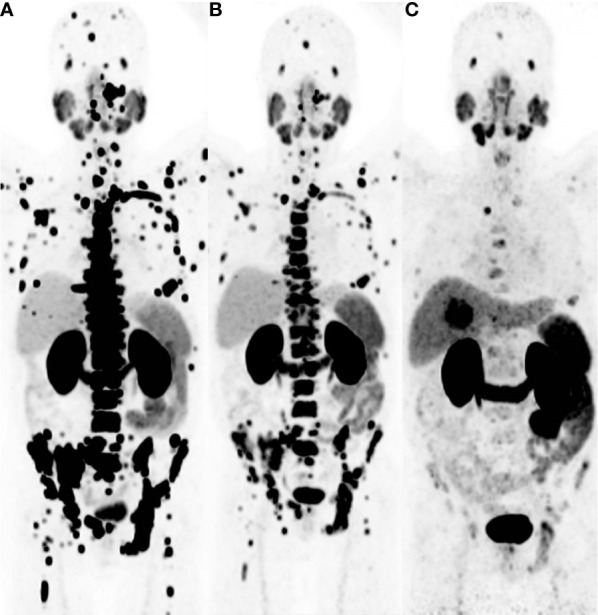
This 63-year-old male patient was diagnosed with prostate cancer 6 years ago and underwent a ^68^Ga-PSMA PET/CT imaging examination. The maximum intensity projection (MIP) image revealed a significant increase in tracer uptake in the skeletal region (A). The patient received 4 cycles of ^225^Ac-PSMA-617 therapy, and after 2 treatment cycles, subsequent ^68^Ga-PSMA PET/CT imaging still showed multiple areas of tracer uptake in the skeletal region, despite a notable reduction in SUVmax (B). A ^68^Ga-PSMA PET/CT imaging performed 16 weeks after the last treatment showed a substantial decrease in tracer uptake in the skeletal lesions (C). Adapted from Ref (82).
